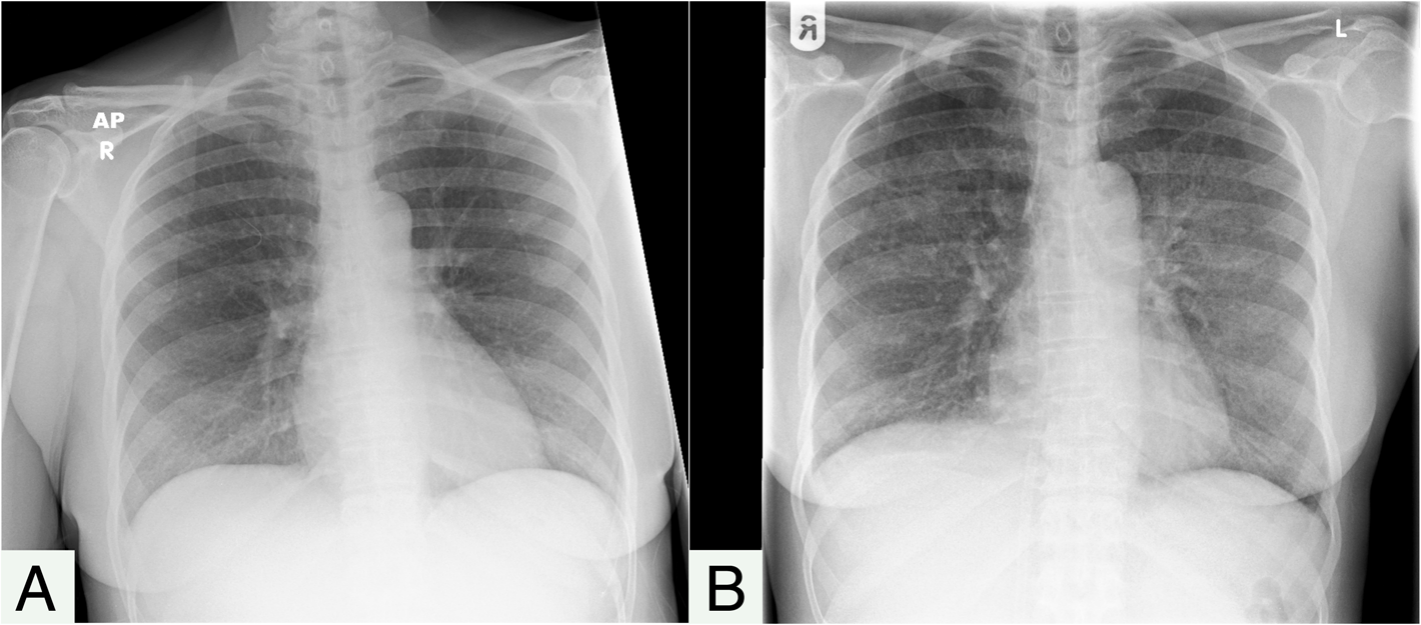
Chest x-ray. a Anterior-posterior (AP) film obtained on admission demonstrating normal heart, lung, mediastinum, and bony thorax shadows. b Posterior-anterior (PA) film obtained on day 4 of admission to investigate the patient’s acute respiratory failure. The film demonstrates extensive new bilateral acinar opacification in a mainly perihilar distribution throughout all lobes of both lungs in a fairly symmetrical distribution. Normal cardiac contours and pulmonary vascularity are seen
